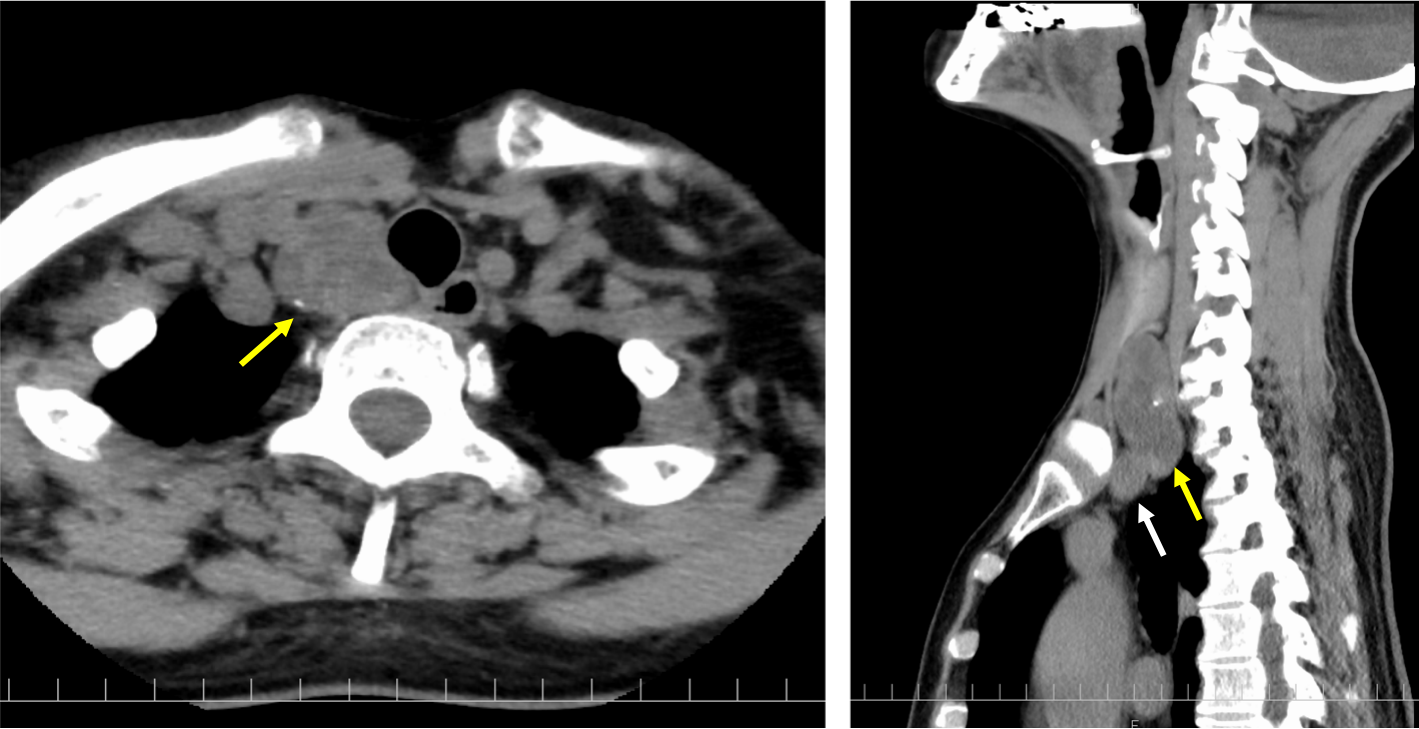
Computed tomography image at cervical extension. The tumor (*yellow arrow*) had extended into the mediastinum and was descending posteriorly into the brachiocephalic artery (*white arrow*)
